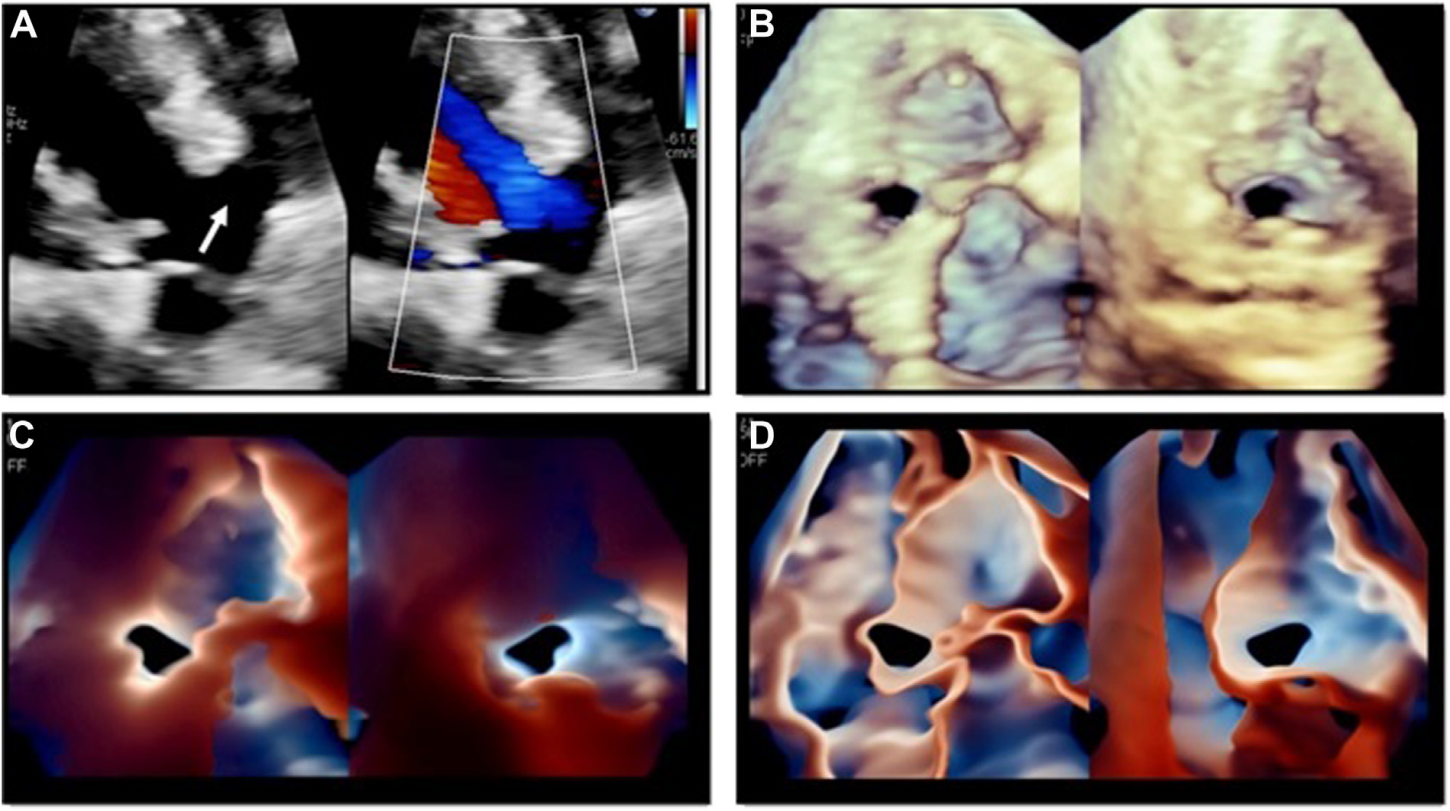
Use of TI in a patient with a diagnosis of membranous VSD. Panel A corresponds to an off-axis TTE parasternal long-axis view where a large membranous VSD can be noticed *(left image, white arrow)*. The *right image* on panel A corresponds to the use of color Doppler in this same view. Panel B corresponds to a 3D full-volume acquisition showing the precise location of the VSD (as visualized from the left ventricle *[left image]* and from the right ventricle *[right image]*). Enhanced border definition of the VSD using TI and Glass renderings are shown in panels C and D, respectively, as seen from the left ventricle *(left images)* and from the right ventricle *(right images)* as well.
